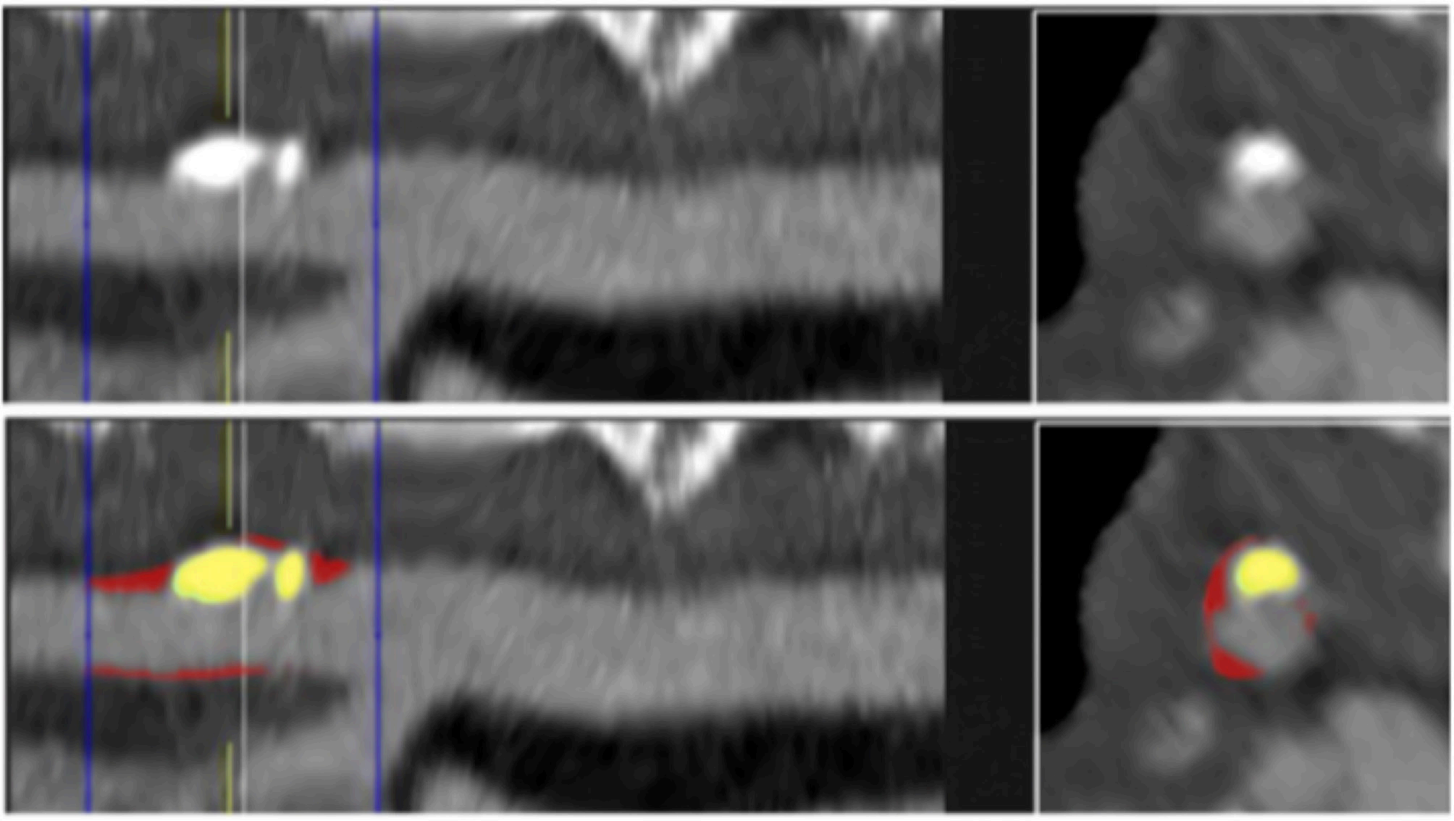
Example of plaque imaging by computed tomography angiogram in the common carotid artery with classification overlay to show non-calcified plaque (red) and calcified plaque (yellow). Reproduced from Ramanathan R, Dey D, Nørgaard BL, *et al.*; Carotid plaque composition by CT angiography in asymptomatic subjects: a head-to-head comparison to ultrasound; *European Radiology*, 2019 (34). Copyright 2019 John Wiley and Sons.
